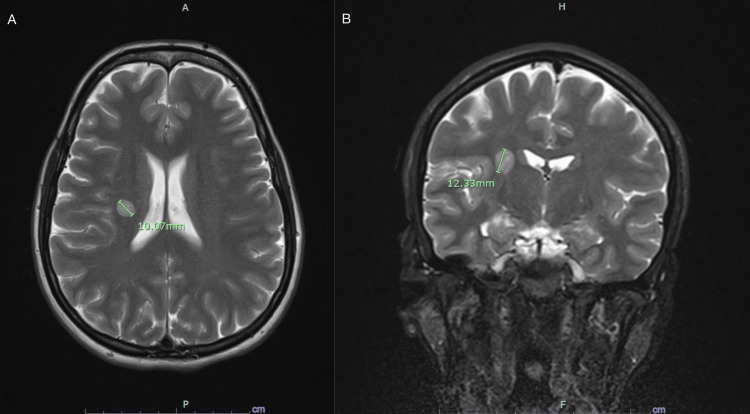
Follow-up MRI four months later Follow-up MRI brain revealing a decrease in size of the previously described lesion in the right corona radiata. Figure 2A displays a T2-weighted axial image showing a decrease in size to 10.07 mm. The coronal view in Figure 2B reveals a decrease in size to 12.33 mm.
